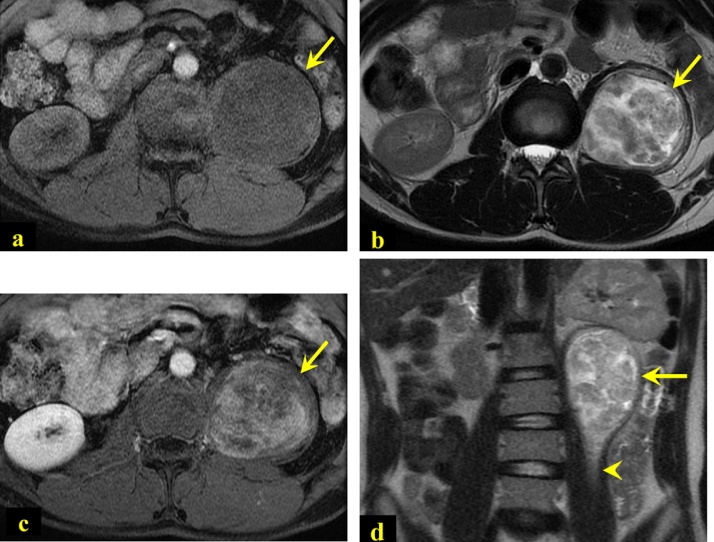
Axial T1 (2a), T2 (2b), and post-contrast T1-weighted (2c) MRI images showed well-defined T1 hypointense, T2 heterogeneous hyperintense, and heterogeneously enhancing mass in the posterior aspect of the left psoas muscle (arrow). Coronal T2-weighted image (2d) showed fat split sign (arrowhead) indicating intramuscular location of the mass.
